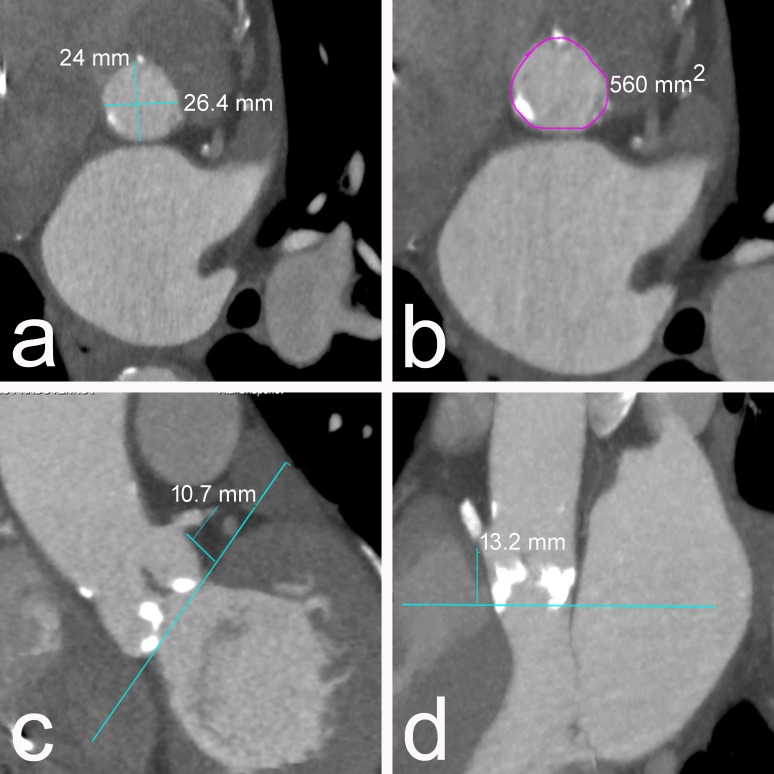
Preprocedural CT evaluation. CT - computed tomography
